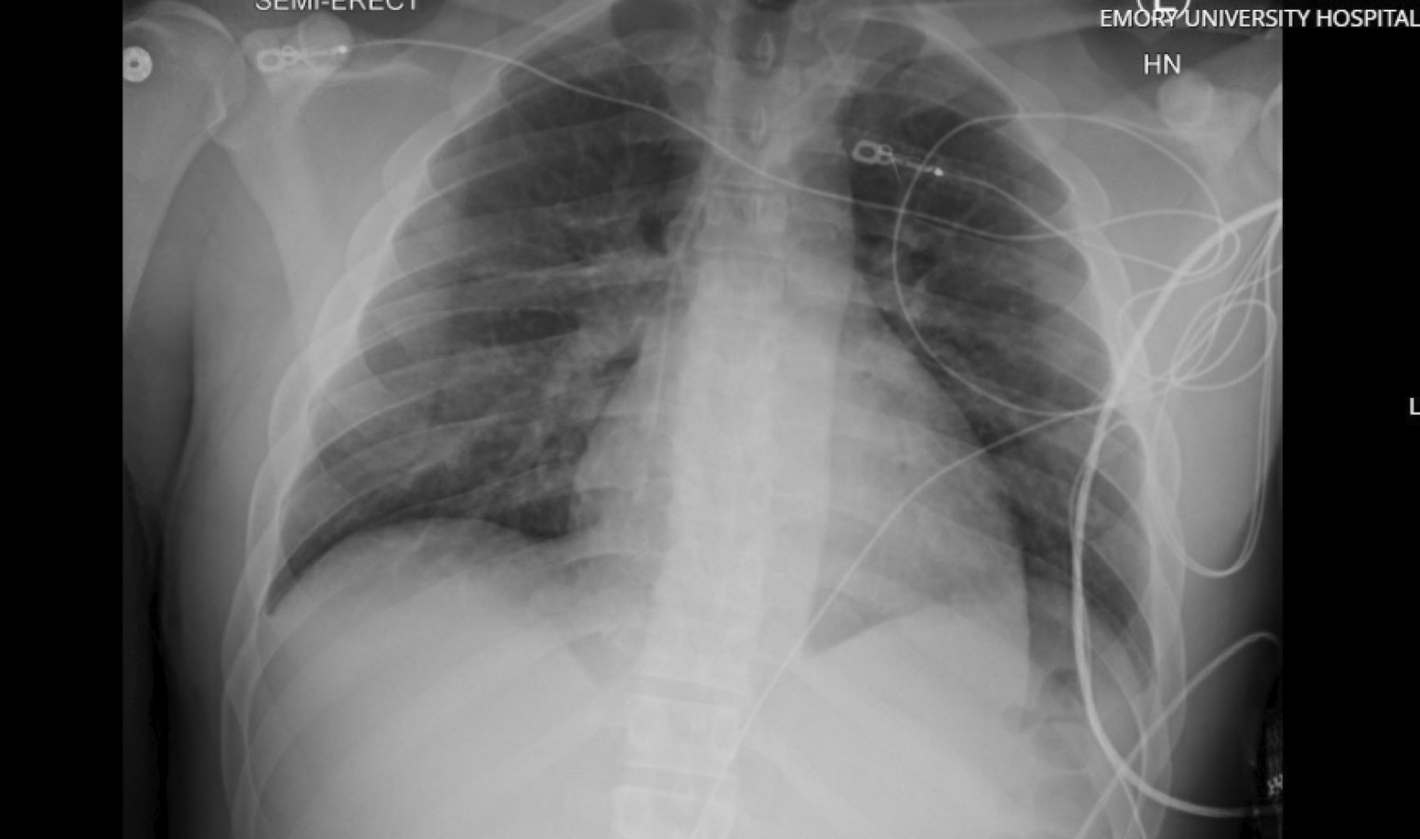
Portable chest radiograph from hospital Day 7 (day before discharge). This radiograph demonstrates interval improvement of pulmonary edema.
